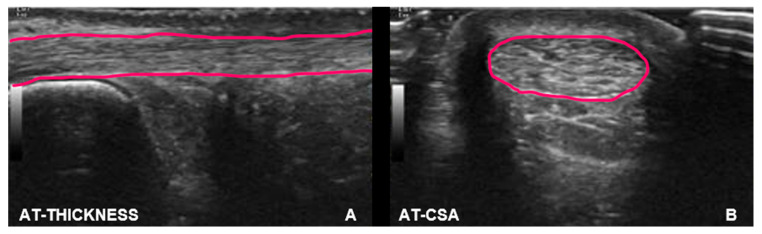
Ultrasound images of AT (i.e., thickness (A) and CSA (B)). Thickness and CSA are highlighted in pink.
